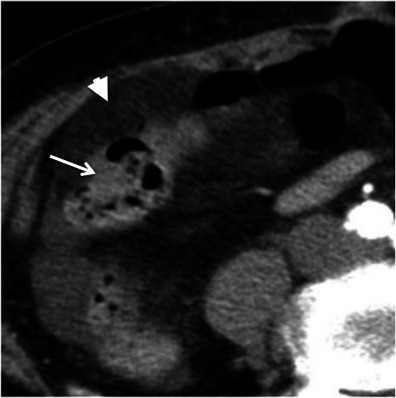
Omental infarction. Axial contrast-enhanced CT scan of a patient who presented with acute right upper quadrant pain shows an inhomogeneous fatty mass (*large arrow*) in the greater omentum, indicative of omental infarction. Note the mild wall thickening (*arrow*) of the adjacent colonic wall, which is clearly disproportionate relative to the fat stranding. *Adapted from the electronic poster “Bowel wall thickening—a complex subject made simple” DOI:*10.5444/esgar2011/EE-063
